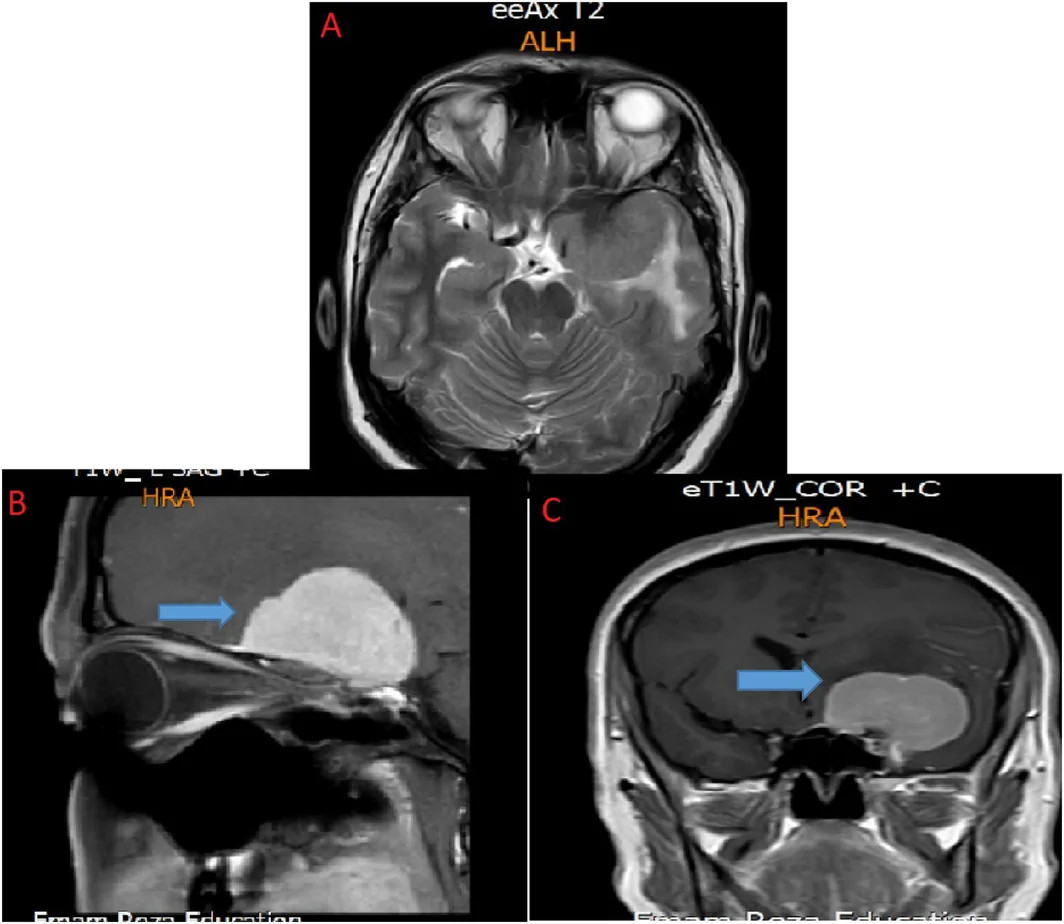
(A) Axial T2 MRI without contrast and left retrobulbar mass. (B) Sagittal T1 MRI with contrast and fat suppression and orbital apex mass enhancement. (C) Coronal T1 MRI with contrast clinoidal enhanced mass (the arrows point to the tumor).
